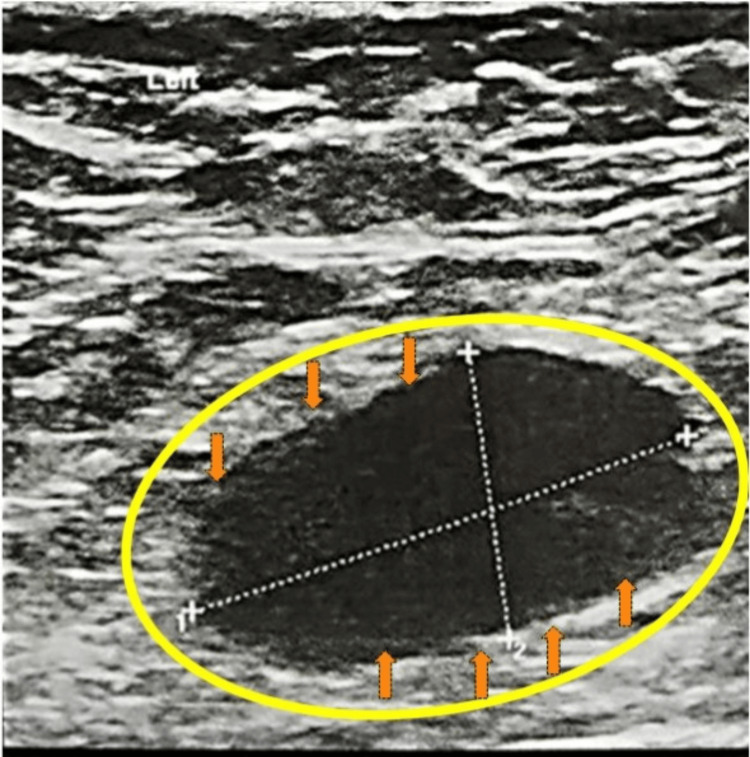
Ultrasound image. The ultrasound image shows an abnormal axillary lymph node (demonstrated with arrows and a yellow circle), which has a lobulated shape and loss of normal echotexture with disappearance of the normal fatty hilum.
